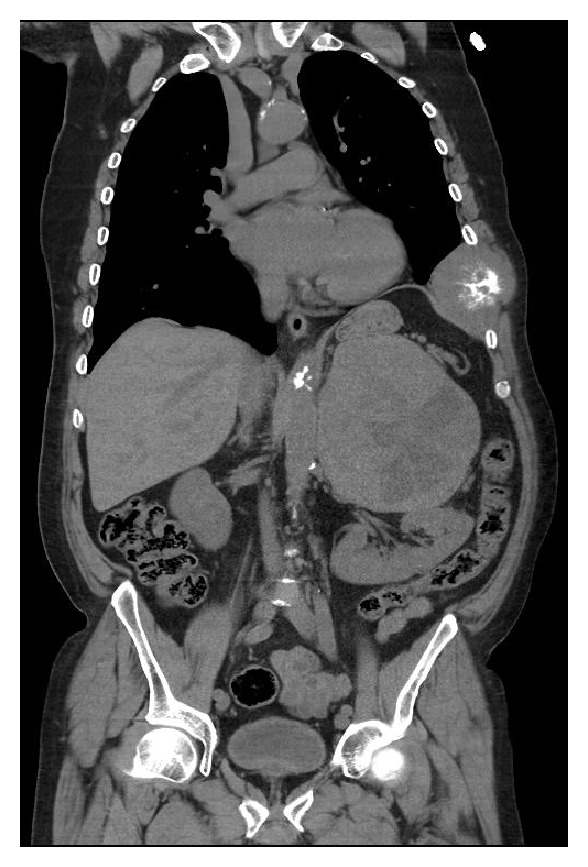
127 × 143 × 144 mm (RL × AP × KK) mass in the left adrenal surgical space, pushing down the left kidney on CT and the rib metastasis.
